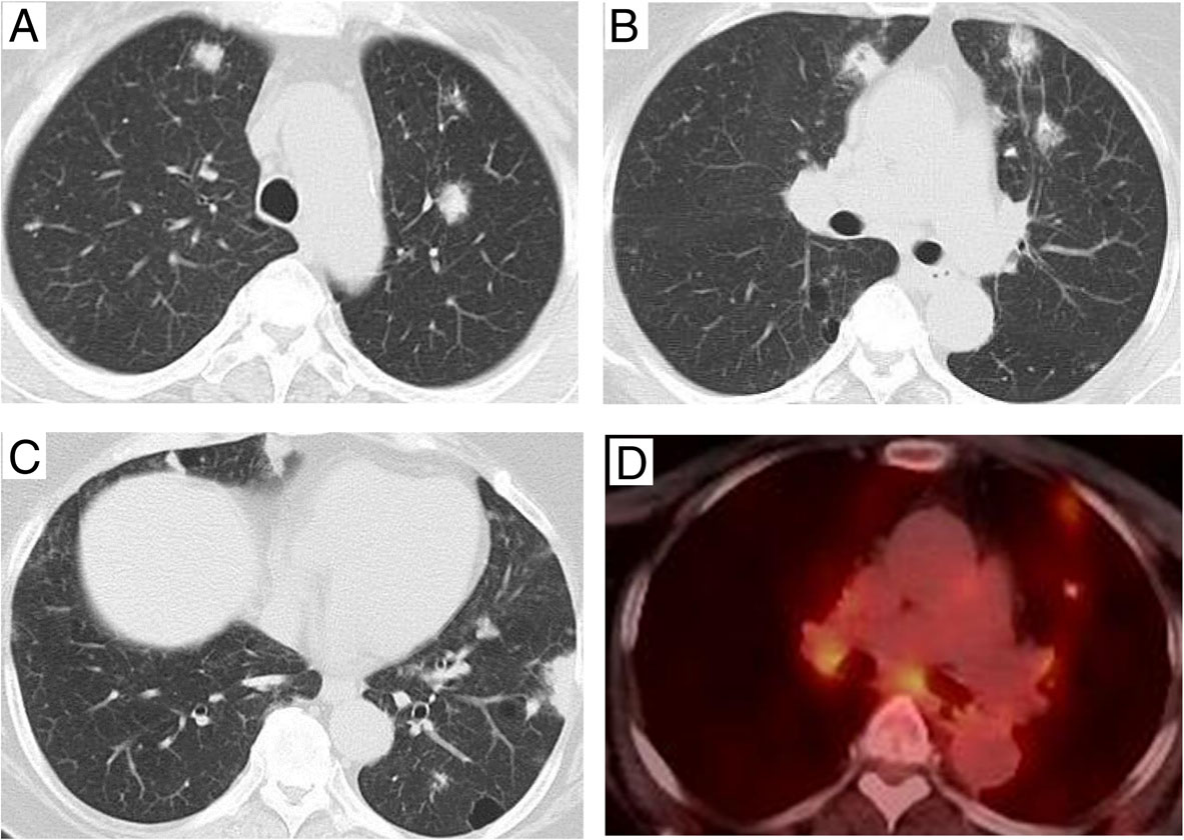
a–c Chest computed tomography showed multiple nodules in the right S3, right S7, left S1 + 2, and left S8. d Fluorodeoxyglucose-positron emission tomography showed FDG accumulation in pulmonary nodules to the horizontal slice as b
